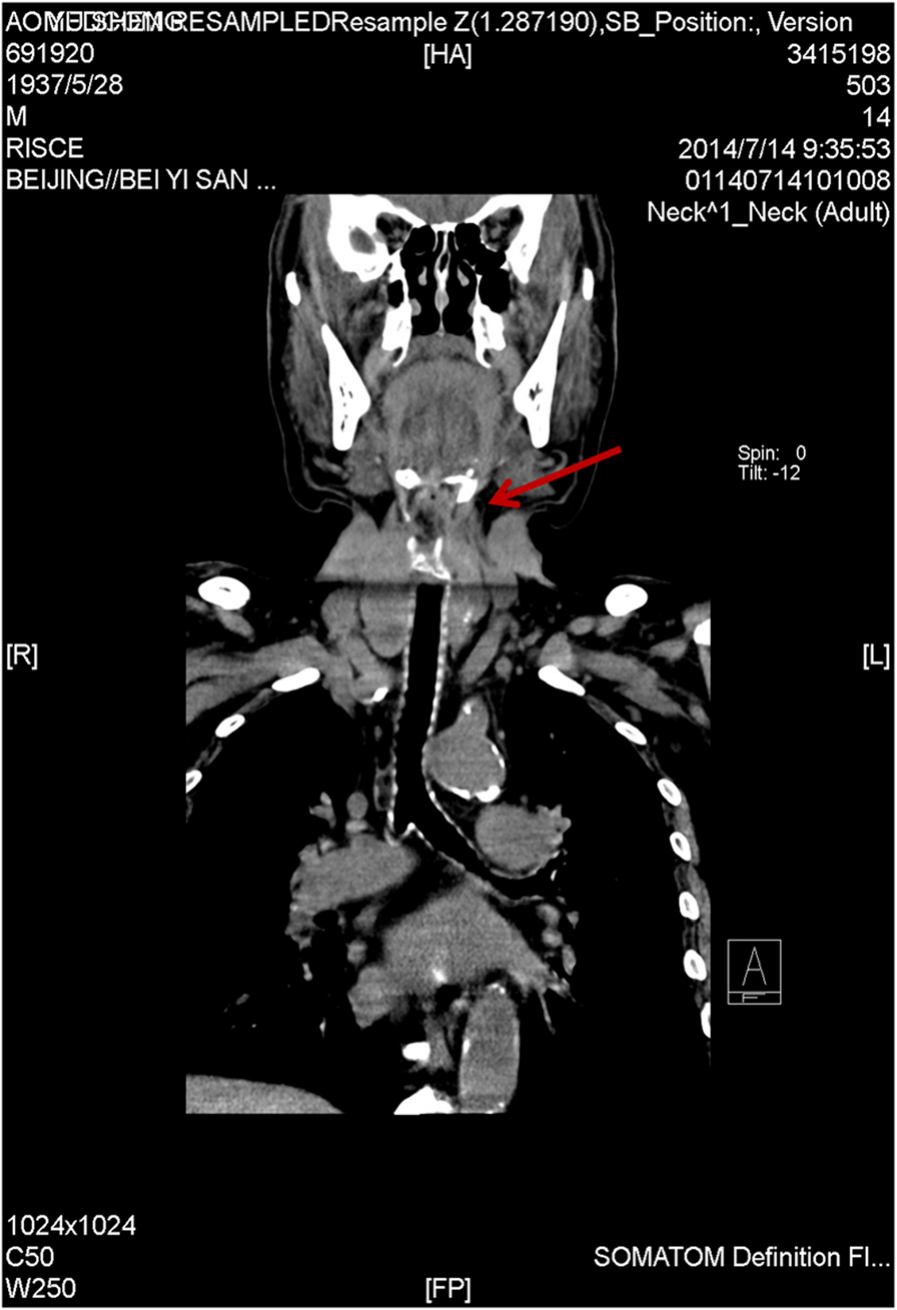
Computed tomography of the neck
